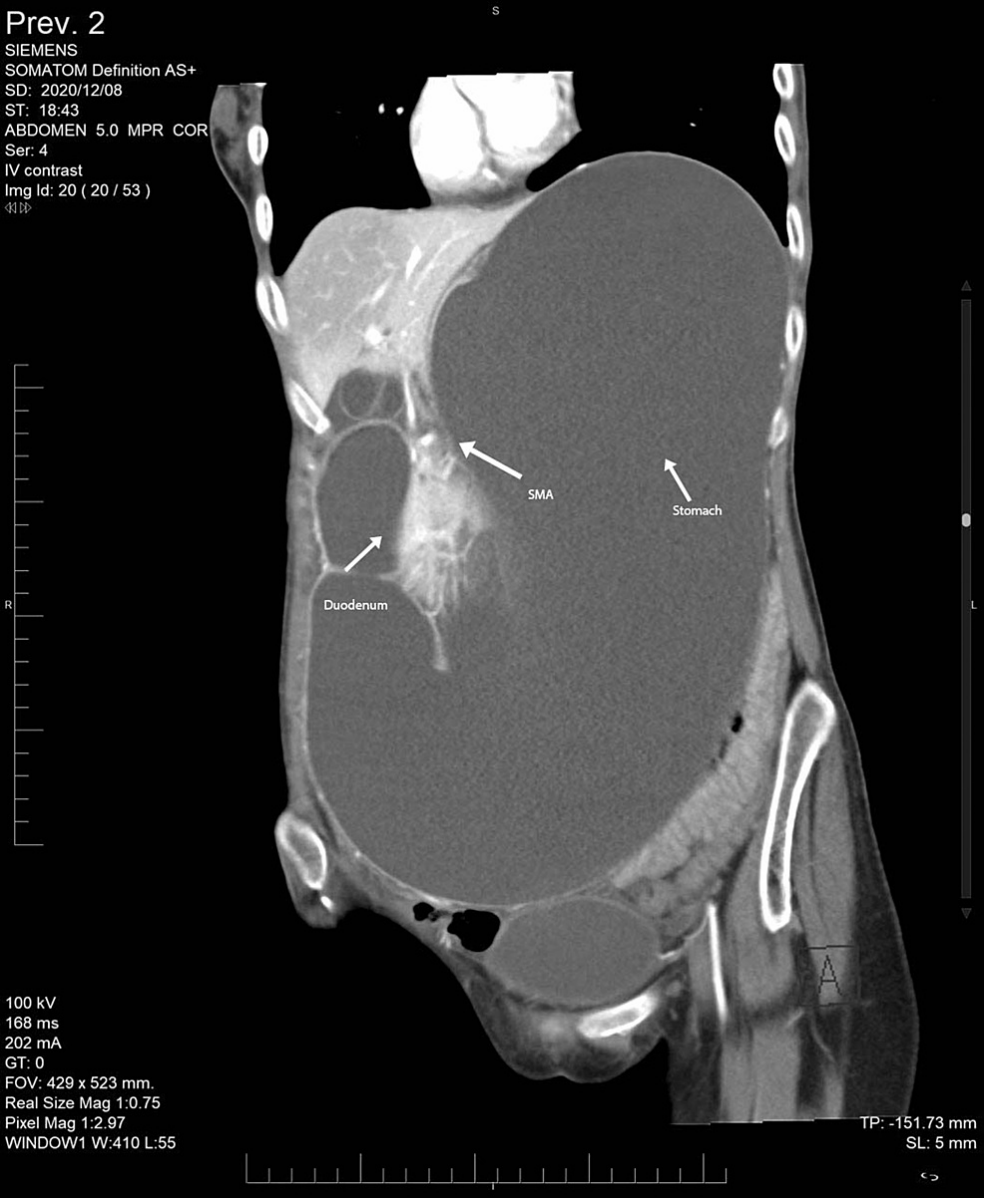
CT showing grossly distended stomach and duodenum. CT: Computed tomography; SMA: Superior mesenteric artery
